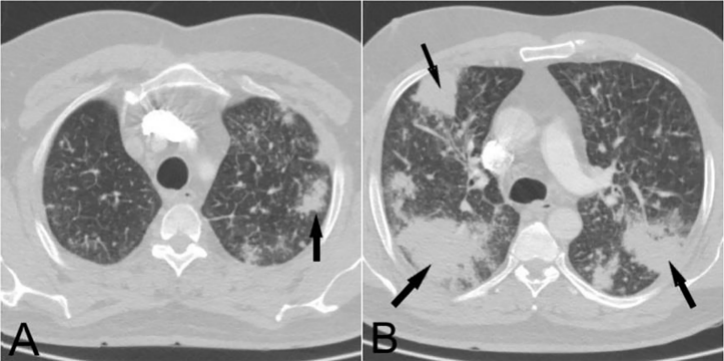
Axial CT scan of a 50-year-old male with a history of cough, dyspnea, and fever for 2months, with no response to antibiotic therapy. Bilateral subpleural consolidations in both lungs are suggestive for COP. Steroid was administered with a significant response to treatment.
